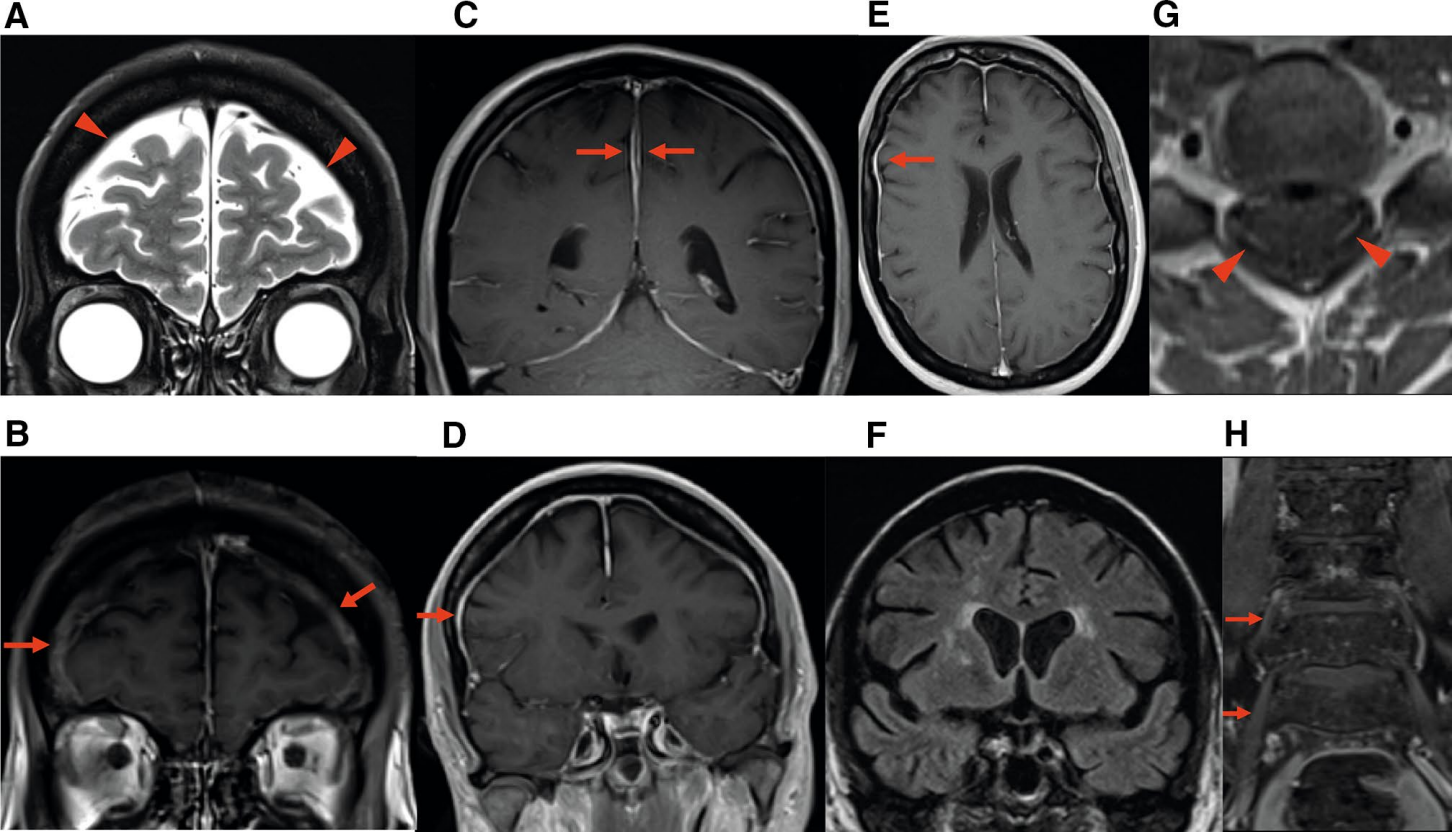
a Coronal T2w image showing apparent widening of subdural planes over bifrontal convexities. b Coronal post-gadolinium T1w equivalent plane image from the same patient demonstrating thick enhancing dura in this area. c Post-gadolinium T1w coronal image showing two opposing layers of enhancing dura. The central non-enhancing area is effusion: the “double falx” appearance. d Post-gadolinium T1w image demonstrating diffuse enhancement of intracranial pachymeninges. e Post-gadolinium T1w image demonstrating focal enhancement of intracranial pachymeninges. f Coronal FLAIR showing moderate degree of small vessel disease. g Post-gadolinium T1w image of the cervical spine level C5 showing intradural leptomeningeal enhancement of cervical nerve roots. h Thickened and enhancing roots/proximal lumbar plexus on post-gadolinium T1w coronal image. For further images and sequences, please see the Supplementary Image Series
